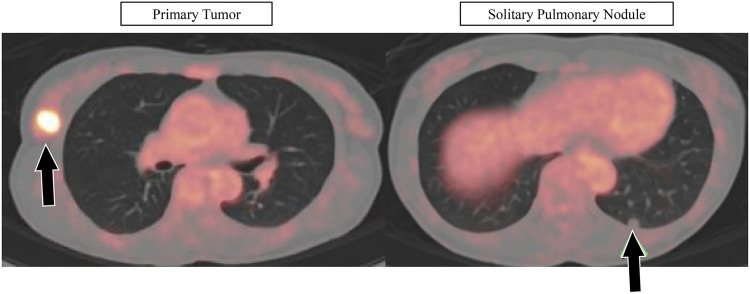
PET-CT findings of the primary tumor and the solitary pulmonary nodule after neoadjuvant chemotherapy (December of Year X). (Left) Image showing strong FDG uptake in the primary breast tumor. (Right) Image of the solitary pulmonary nodule in the left lower lobe showing no abnormal FDG uptake (SUVmax <2.5). Arrows indicate the primary breast tumor on the left image and the location of the solitary pulmonary nodule in the left lower lobe on the right image. FDG, fluorodeoxyglucose; SUVmax, maximum standardized uptake value
